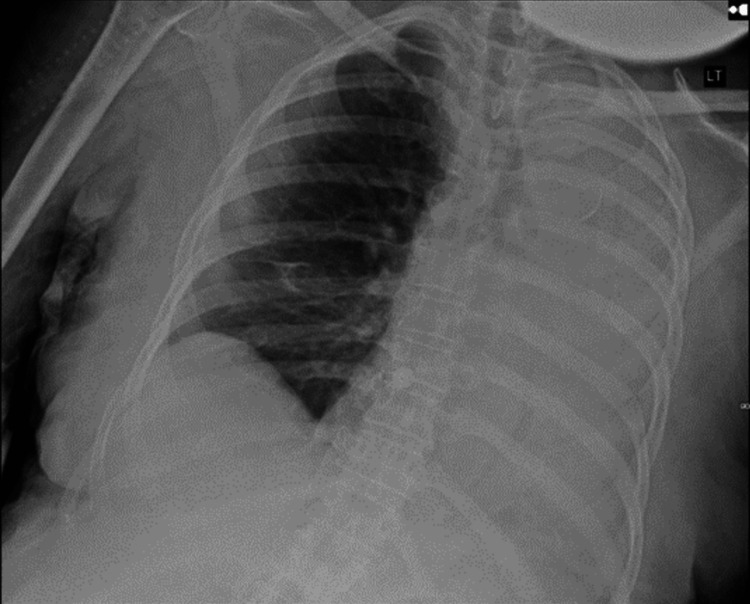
Anteroposterior chest x-ray five days post-admission. Complete opacification of the left lung field. CXR, chest x-ray
